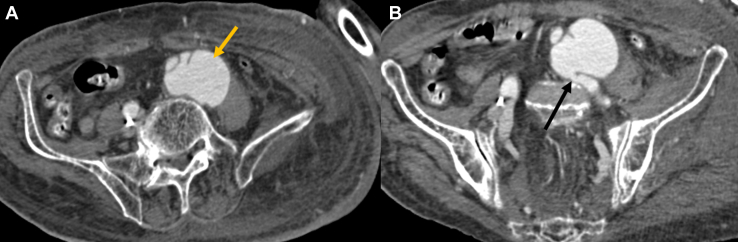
A CT angiography revealed a saccular aneurysm of the left common iliac artery (yellow arrow), with a fistulous communication to the left common iliac vein (black arrow). CT, computed tomography.
